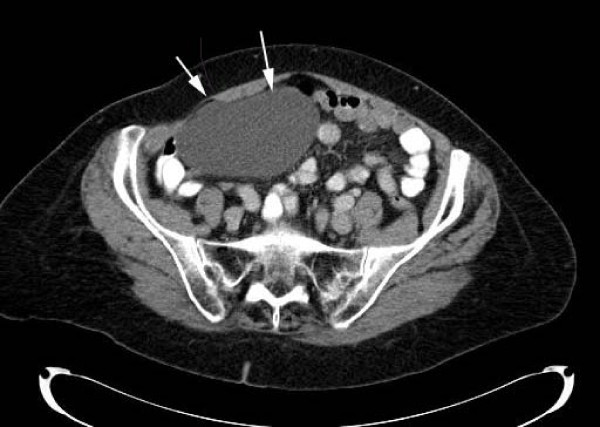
Abdominal CT scan. (Arrows indicate boundaries of cyst).
